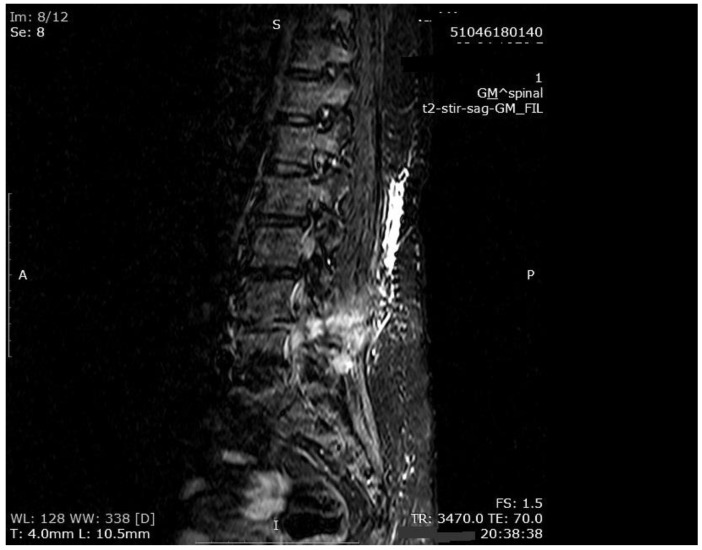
MR imaging T2-weighted scan showing inflammatory infiltrates in the course of pre-HBO_2_ treatment.
